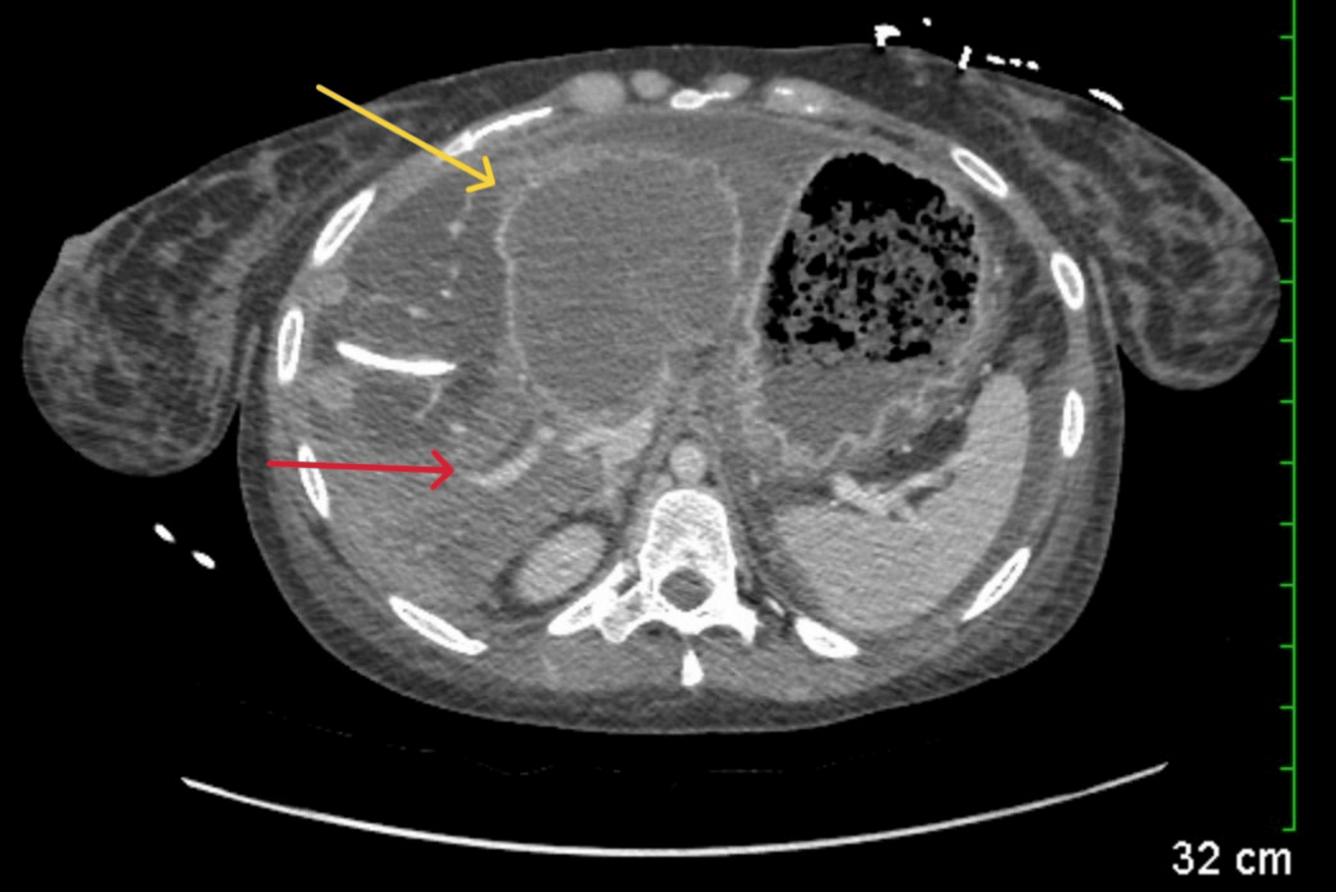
Abdominal CT with IV contrast showing multiple peripherally enhancing masses within the liver (yellow arrow), as well as bilateral intrahepatic biliary ductal dilation (red arrow) CT, computed tomography; IV, intravenous
